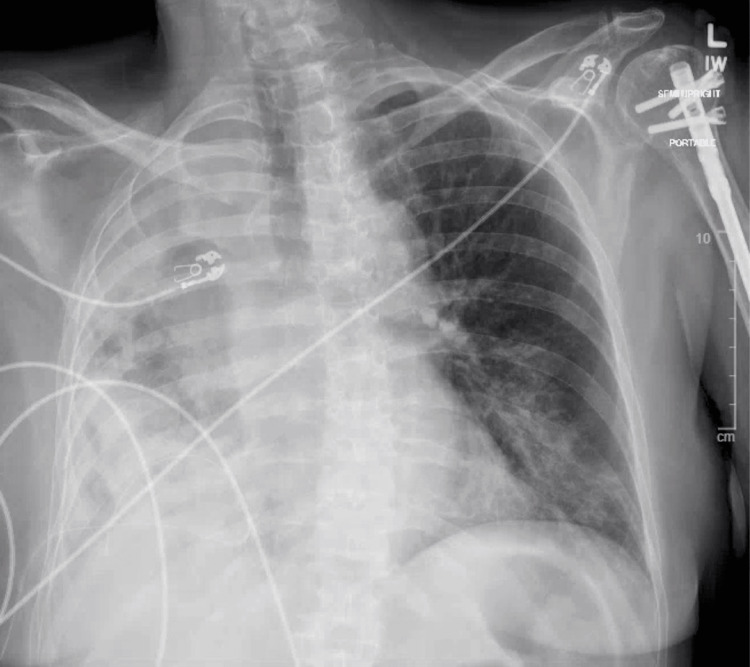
Chest X-ray demonstrating dense right-sided lung consolidation consistent with large malignant pleural effusion.
